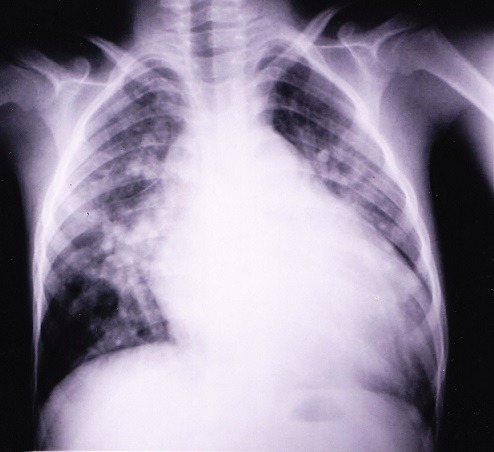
Radiographie pulmonaire de face montrant l’accentuation de la vascularisation pulmonaire
